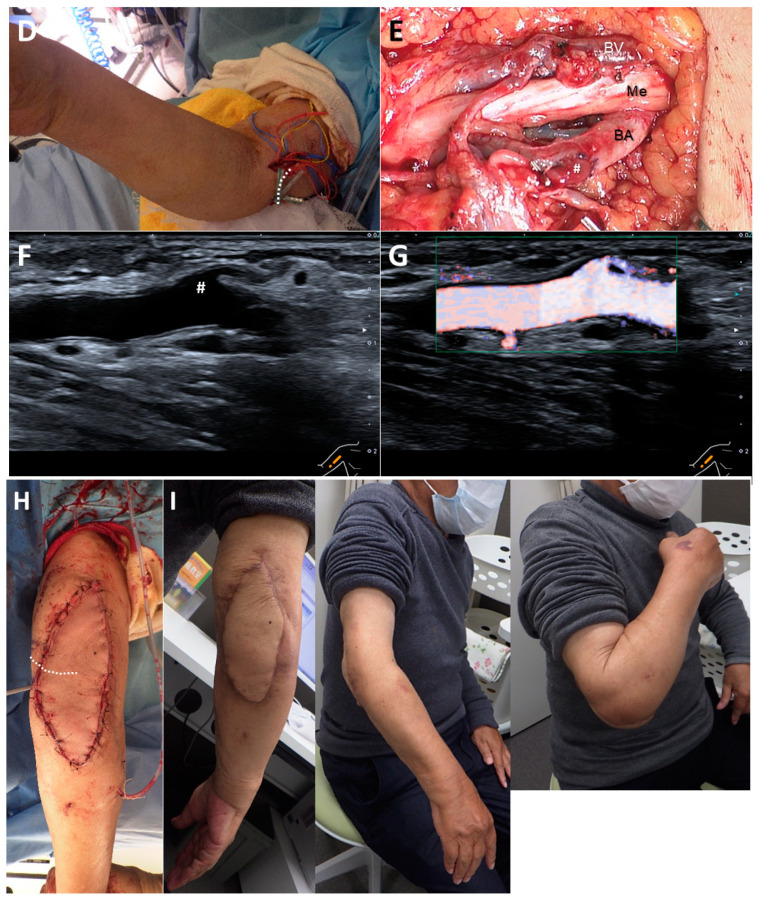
Representative case (Case 6). The patient is a 74-year-old male with rheumatoid arthritis. (A): Four years after total elbow arthroplasty, the patient developed an infection. (B): Plain radiographs show no evidence of implant loosening. (C): Despite multiple debridements and antibiotic therapy, which successfully controlled the infection, a large soft tissue defect developed on the posterior aspect of the elbow. (D): On the 14th day after the initial surgery, a free ALT flap was performed using the BA as the recipient vessel (flap size: 90 cm^2^). The flap pedicle vessels (dotted line) were guided through a spacious subcutaneous tunnel to the anterior aspect of the BA. (E): The flap pedicle vessels were aligned parallel to the BA and accompanying brachial vein (BV), ensuring a smooth transition towards the defect site (BA diameter: 5.5 mm, flap pedicle diameter: 1.5 mm). The anastomosed artery (hashmark) and vein (asterisk) can be seen bulging over the BA and accompanying vein. (Me; median nerve). (F): Ultrasound imaging at three weeks postoperatively shows a well-expanded anastomosis (hashmark). (G): Color Doppler imaging reveals no turbulence at the anastomosis site, with adequate blood flow in both the flap vessels and the distal recipient vessels. (H): The soft tissue defect is adequately covered by the ALT flap. (I): No postoperative complications were observed, and at the final follow-up one year after surgery, the patient had an elbow extension of −20° and flexion of 145°, with a “Good” outcome.
